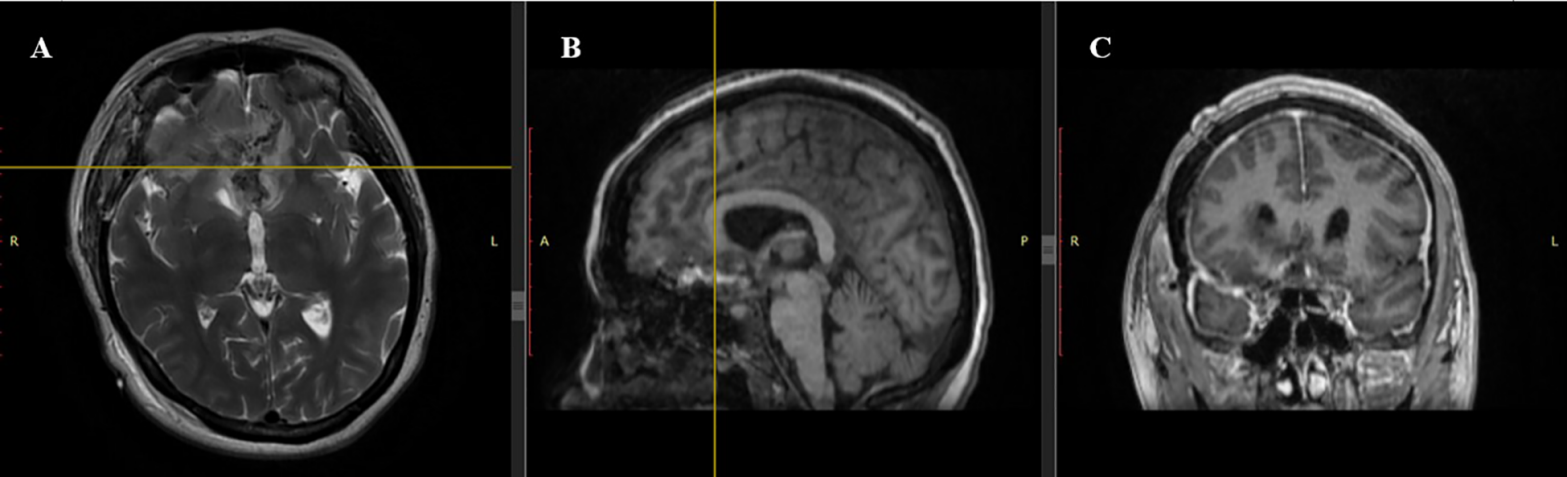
Postoperative MRI scan. (A) Axial T2-weighted sequence: Complete resection of the tuberculum sellae meningioma with restored anatomy of the frontal lobes. Minimal postoperative changes are observed without significant edema. (B) Sagittal T1-weighted sequence: Hypointense resection cavity with no visible residual tumor. Midline structures are realigned, reflecting resolution of preoperative displacement. (C) Coronal T1-weighted sequence: Symmetry of frontal lobes restored with no residual tumor, hematoma, or cerebrospinal fluid accumulation.
